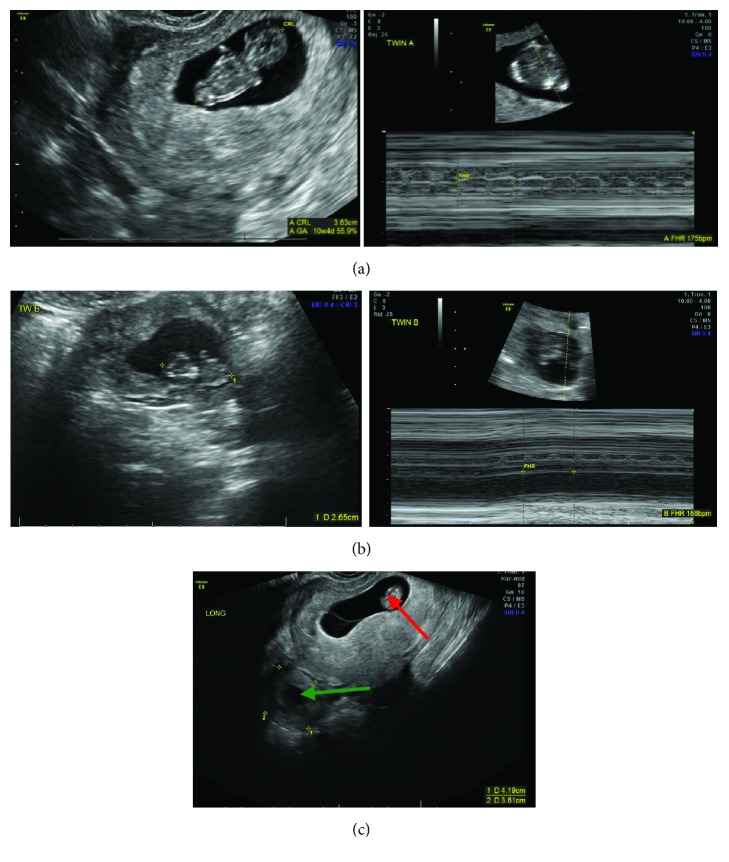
Official ultrasound showing positive FHR for Twin A, intrauterine pregnancy (IUP) (a); for Twin B, right tubal ectopic pregnancy (EP) (b); and presence of both Twin A [intrauterine pregnancy (IUP)] (red arrow) and Twin B [ectopic pregnancy (EP)] (green arrow) (c).
